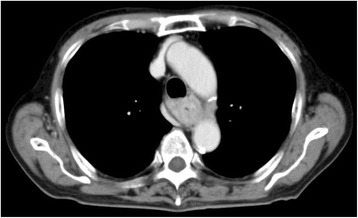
Computed tomography of the chest: An esophageal tumor, which appears to compress the membranous portion of the trachea, is considered to be indicative of advanced esophageal cancer as an *en bloc* mass with lymph node metastasis
